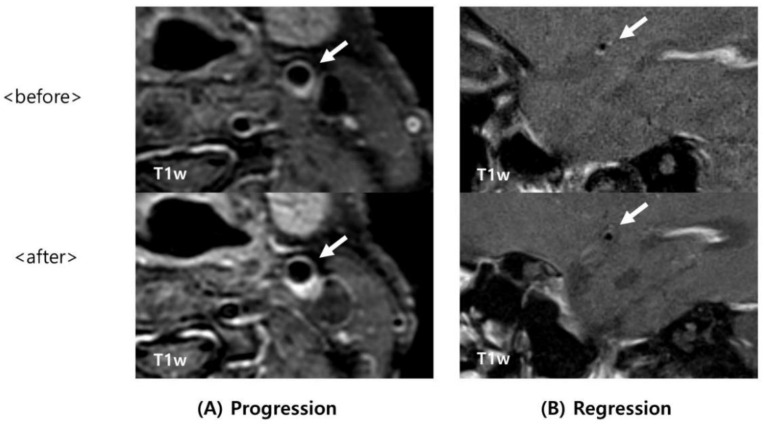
Examples of progression and regression of intraplaque hemorrhage on HR-MRI. (A,B) Atherosclerotic plaque with intraplaque hemorrhage (arrow). (A) Upper panel: initial T1-weighted (T1w) HR-MR imaging in the left CCA shows an eccentric plaque with intraplaque hemorrhage (IPH); lower panel: follow-up T1w HR-MR imaging shows significant atherosclerotic lesion with high signal intensity in the same area that indicates progression of IPH more than the initial imaging does. (B): Upper panel: initial T1w HR-MR imaging shows an eccentric lesion with IPH in the MCA. Lower panel: follow-up T1w HR-MR imaging reveals the MCA without IPH. HR-MRI = high-resolution magnetic resonance imaging; CCA = common carotid artery; MCA = middle cerebral artery.
